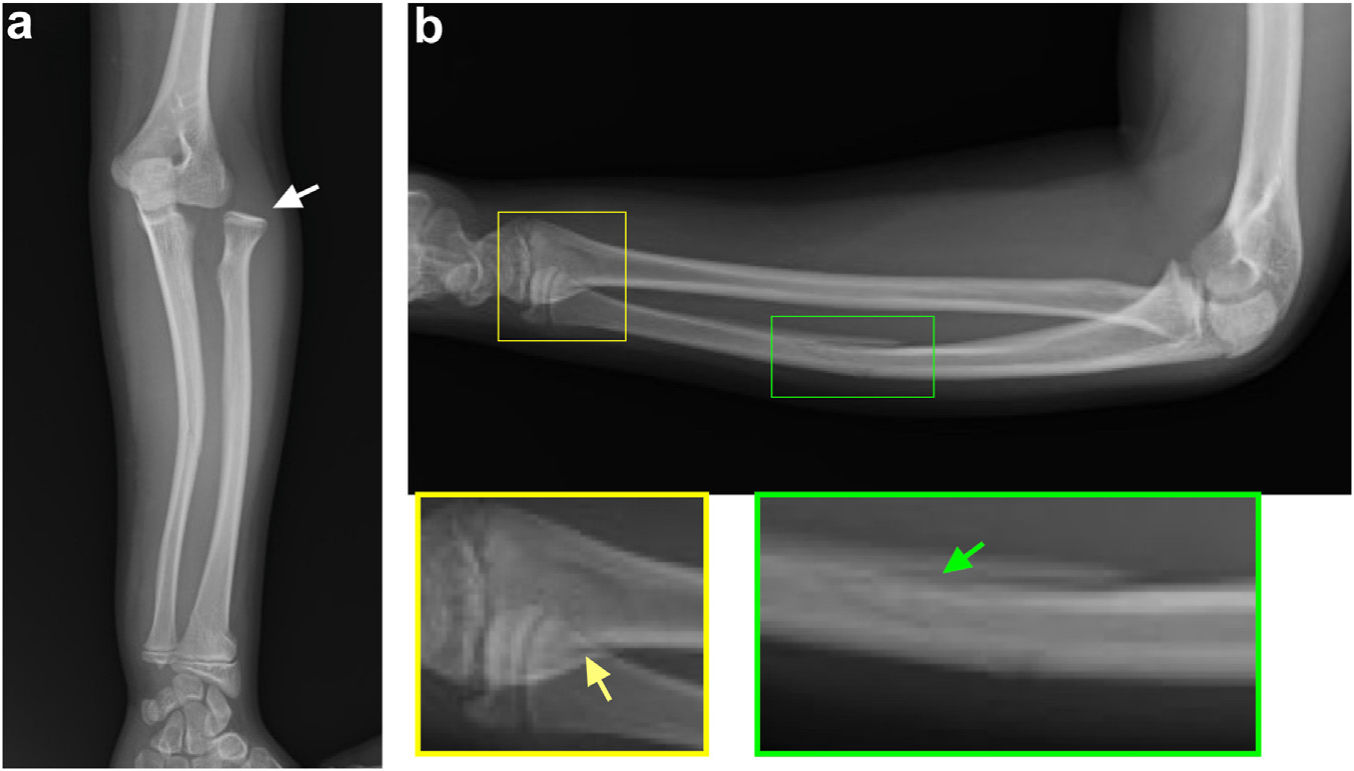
Initial radiographs. (a) Antero–posterior view displaying lateral dislocation of the radial head (*white arrow*). (b) Lateral view revealing minimally displaced fractures of the distal radius (*yellow arrow*) and ulna shaft (*green arrow*).
